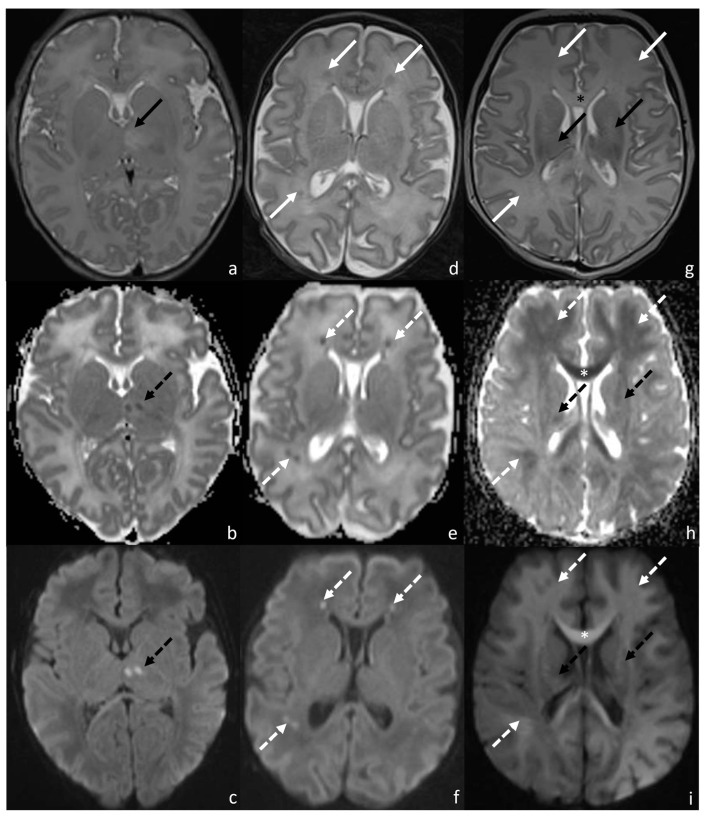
MRI0 of patients affected by HIE injury and presenting: pattern 1 (a–c), characterized by the prevalent involvement of basal ganglia, thalami, and perirolandic areas; pattern 2 (d–f), characterized by the prevalent involvement of watershed areas (c,d); pattern 3, characterized by mixed patterns (g–i). In particular, the figure shows: T2WI (a), ADC (b), and DWI (c) sequences of patients presenting with pattern 1 and showing areas of diffusion restriction in the left thalamus (dotted black arrow in (b,c)), paired with focal left thalamic hyperintensity in T2 (black arrow in (a)); T2WI (d), ADC (e), and DWI (f) sequences of patients presenting with pattern 2 and showing areas of diffusion restriction in the left and right frontal and right parietal white matter (dotted white arrows in (e,f)), paired with inhomogenous T2 hypointensity in the same areas (white arrows in (d)); T2WI (g), ADC (h), and DWI (i) sequences of patients presenting with mixed patterns showing both areas of diffusion restriction in the capsulo-lenticular regions bilaterally (dotted black arrows in (h,i)), which match with the focal areas of T2 hyperintensity in the same areas (black arrows in (g)); and diffuse areas of diffusion restriction in the corpus callosum (white * in (h,i)) and in the left and right frontal and right parietal white matter (dotted white arrows in (h,i)), with aT2 hyperintensity match in the corpus callosum (black * in (g)) and no clear evidence of T2 signal alterations in the remaining areas of (g).
